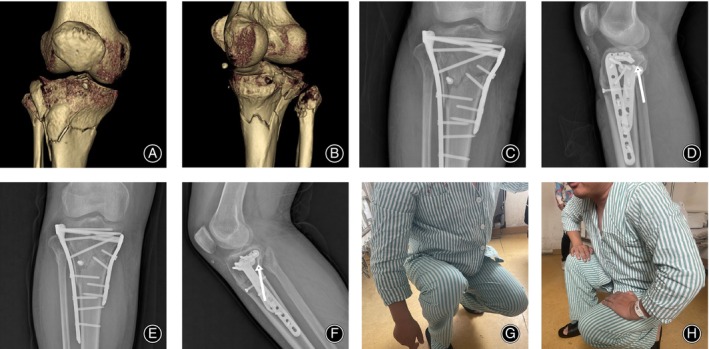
Negative indicator associated with poor functional outcomes by a three‐column fractured case treated by the dual‐plate method. (A, B) The preoperative three‐dimensional CT image revealed that the three columns were all involved. (C, D) Postoperative X‐rays showed that the dual‐plate method could not maintain the anatomical reduction and the arrow signified a backward posterior tibial slope which could not be restored anatomically even though an anteroposterior screw has been applied. (E, F) The backward posterior slope was obvious at the finial follow‐up (14 months). (G, H) The limited knee flexion at the final follow‐up and the patient went for the hardware removal owing to frequent pain and muscle strength weakness, especially during bending.
